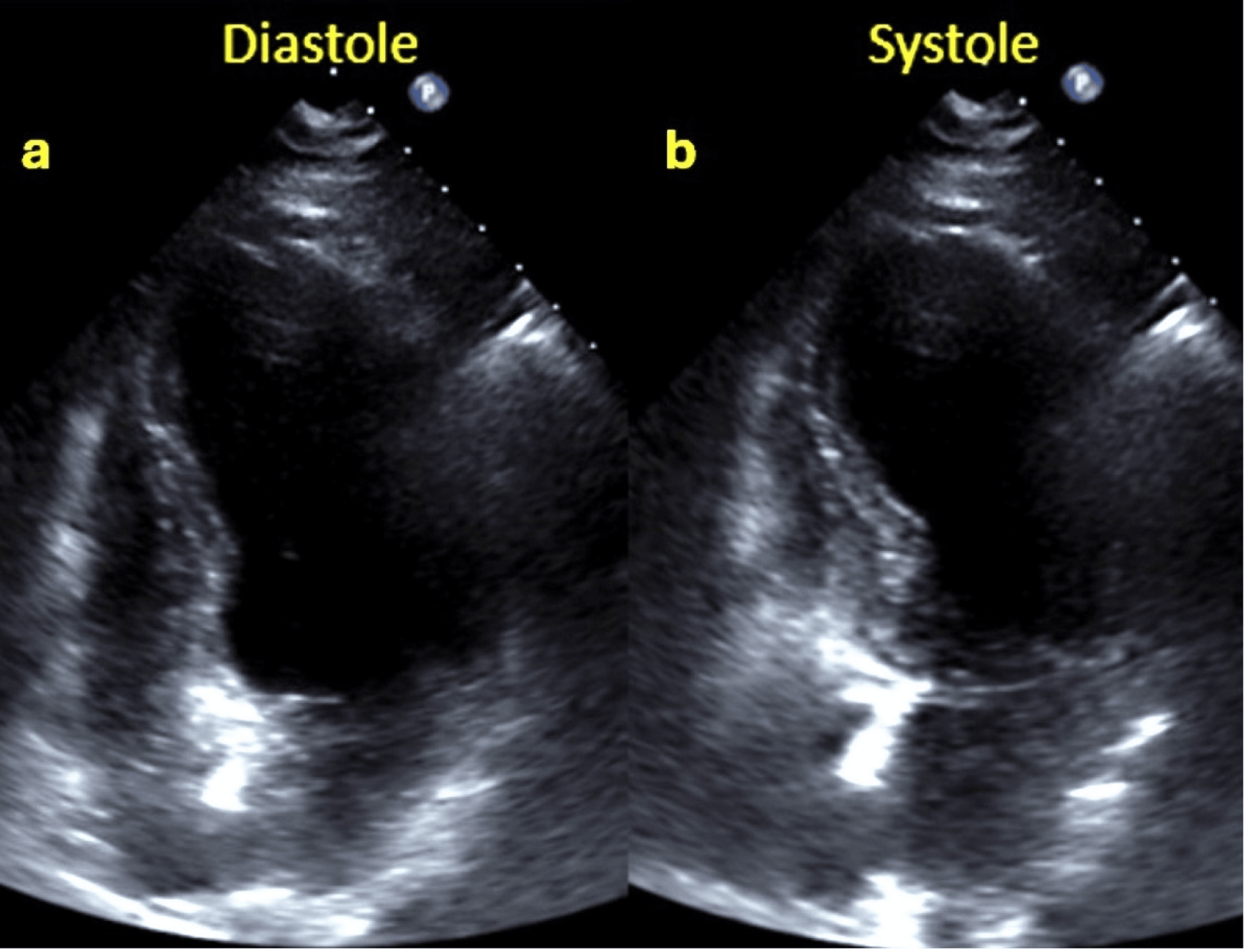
Echocardiogram after PCI shows moderate left ventricular systolic dysfunction and akinesia of the middle and apical segments of all sides a: LV diastole apical four-chamber view; b: LV systole apical four-chamber view showing akinesia of the middle and apical segments of all sides PCI: Percutaneous coronary intervention, LV: Left ventricle
